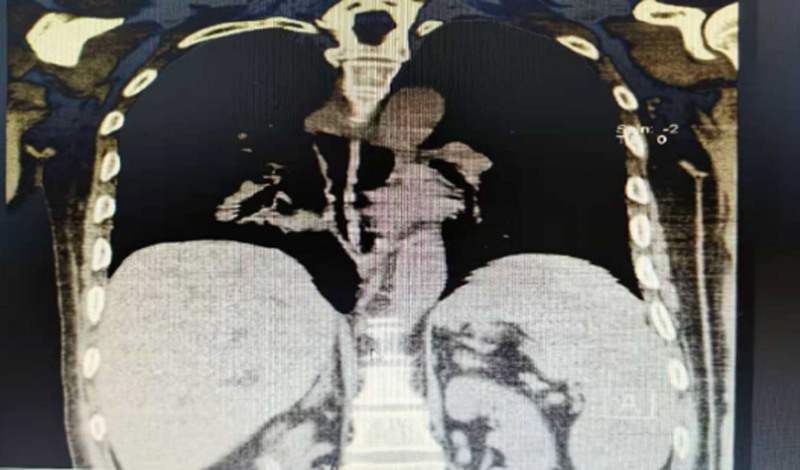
Coronal chest CT: follow-up chest CT scan demonstrated the disappearance of the foreign body in the esophagus, accompanied by significant esophageal dilation. CT = computed tomography.
